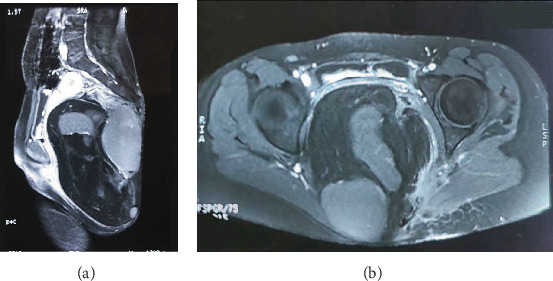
(a) Magnetic resonance imaging (MRI) showing a large well-defined mass with mixed solid and cystic component in lower sacral region. (b) MRI showing multiloculated cystic lesion causing anterior displacement of uterus.
